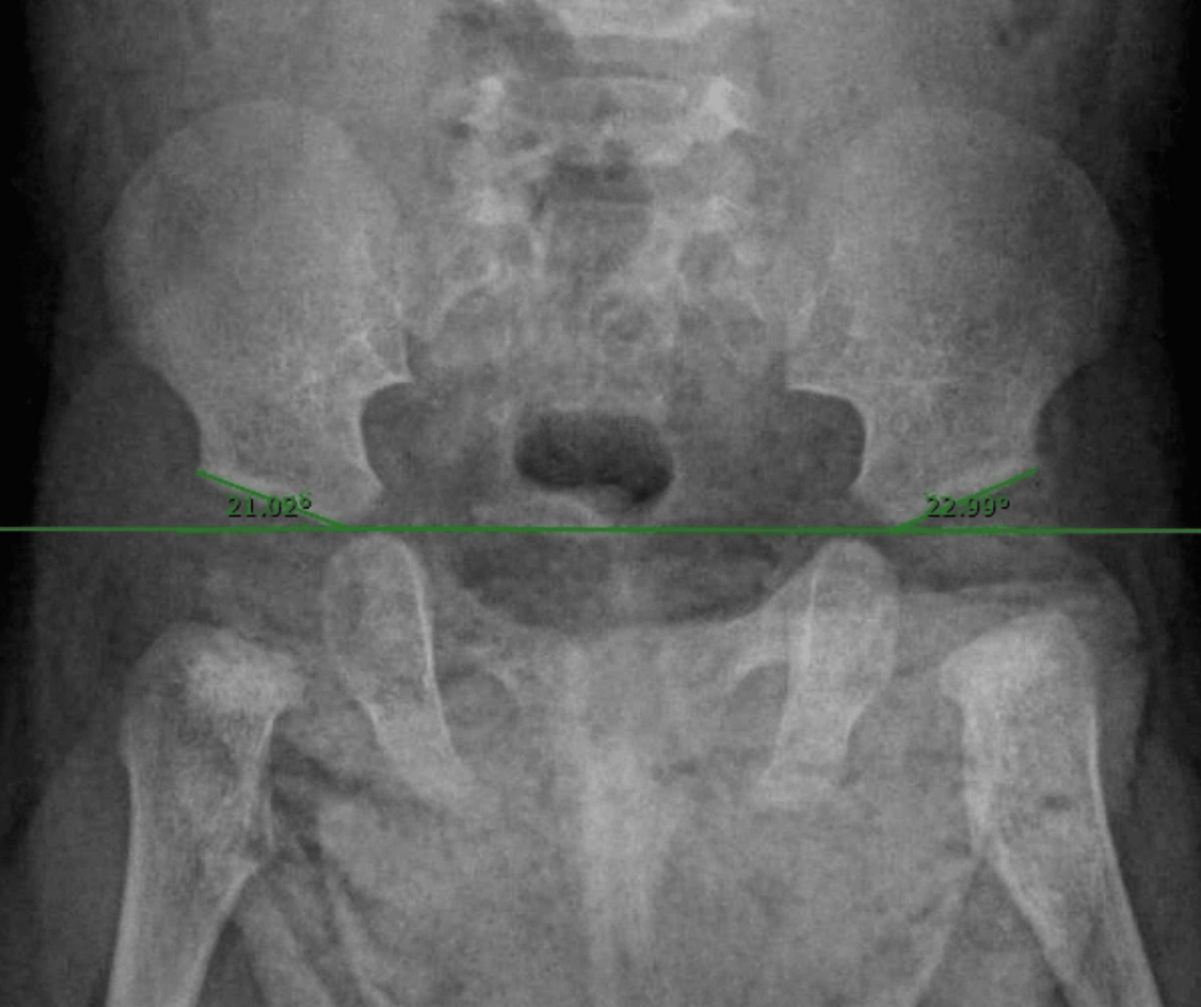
Radiographic image of the hip showing acetabular index measurements for both hips
